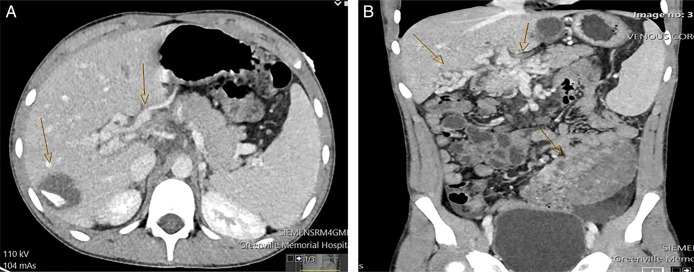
(A) Axial portal venous phase computed tomography (CT) scan showing calcification within the liver cyst right lobe (arrow) and cavernous transformation of the portal vein. (B) Portal venous phase coronal CT scan showing massive varices within the gallbladder wall and at the porta hepatis (arrows) as well as enhancement of markedly enlarged vessels within the very thickened sigmoid colon.
